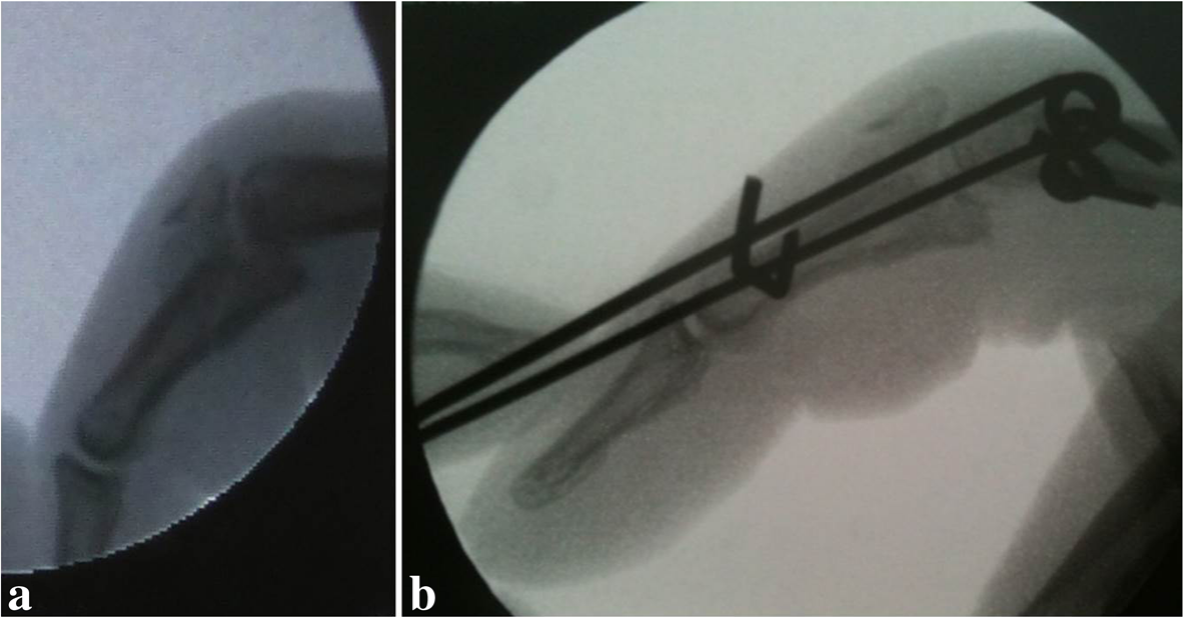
A patient with unstable index finger proximal interphalangeal joint (PIPJ) dorsal lip fracture dislocation. a Lateral view X-ray. b Intraoperative imaging to ensure fracture reduction and PIPJ space
